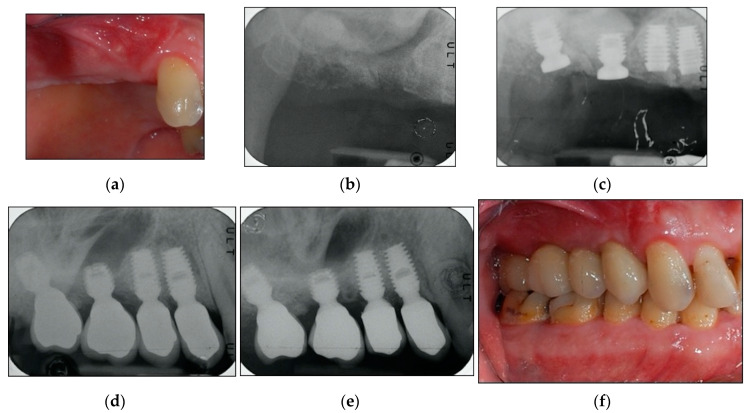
(a–f). Clinical case: Four implants placed in 1.4, 1.5, 1.6, and 1.7 sites. (a) Clinical photograph before implants placement. (b) Pre-operative radiograph before implants placement in sites 1.4, 1.5, 1.6, and 1.7. See minimal bone levels. (c) Radiograph obtained at time of implants placement. Two of the implants have sinus lift abutments. Augmented sinus visible. (d) Implants restored. Radiograph obtained at time of loading. (e) Radiograph obtained at the three-year follow-up. See stable bone levels both for sites with augmented sinus floor and for sites without it. (f) Clinical photograph at three-year follow-up. See stable clinical conditions.
